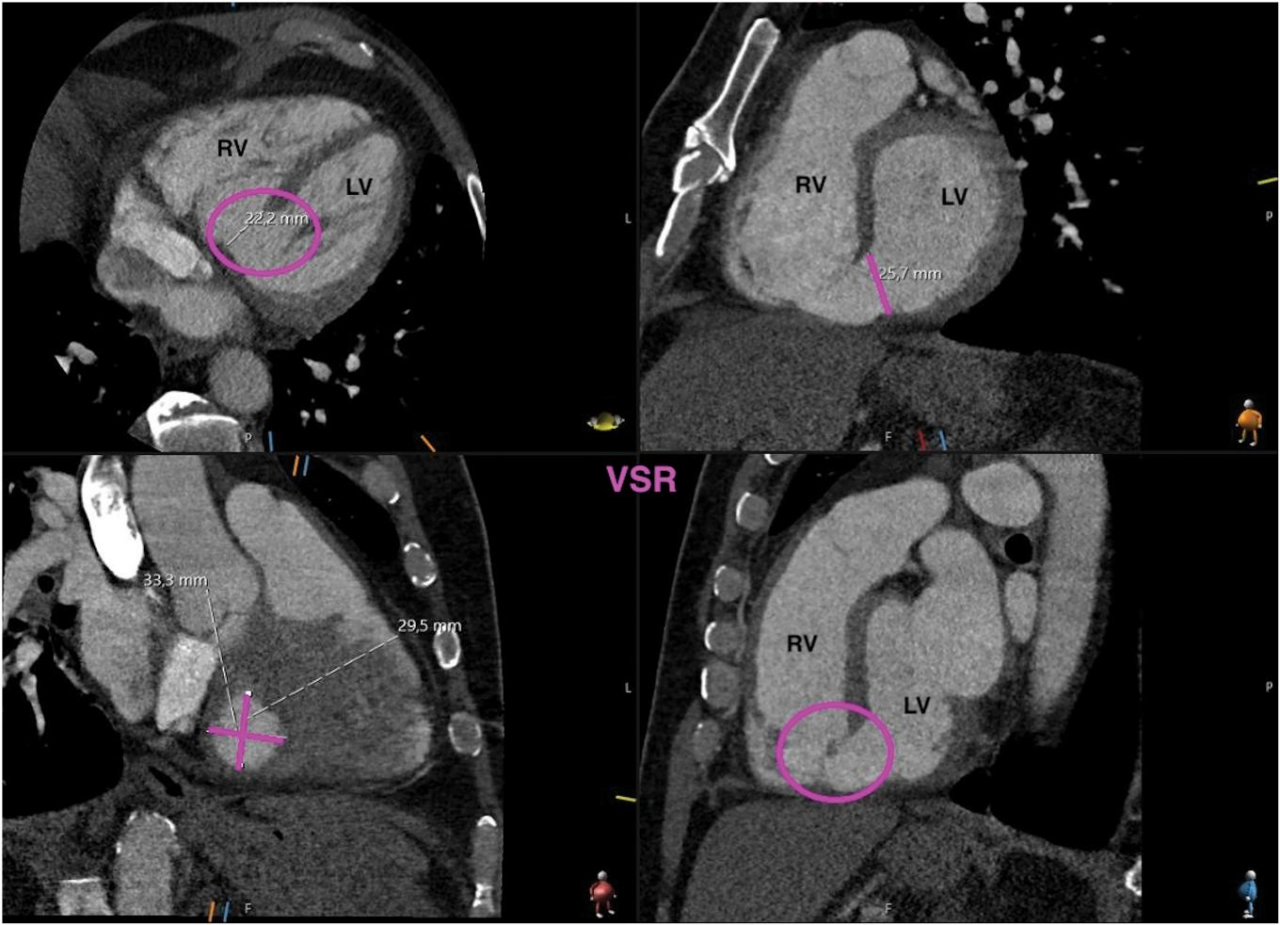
Preoperative computed tomography scan of the post-infarction ventricular septal rupture. Different views in computed tomography scan, with measurement of the VSR; VSR, ventricular septal rupture.
